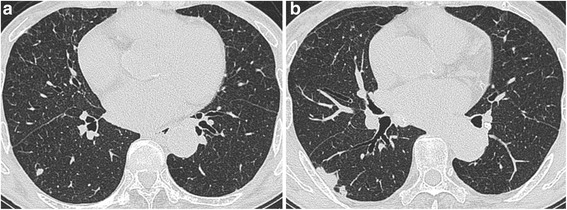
Chest CT showed that a right S6 small nodule was increased (a) and new multiple nodules appeared in the right lower lobe (b)
